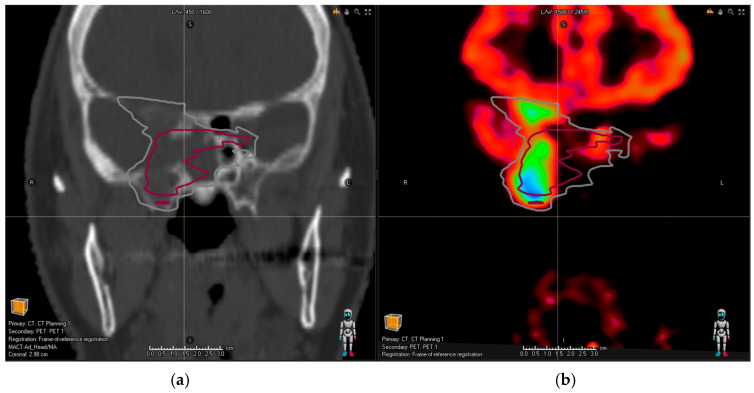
Planning CT image (a) of a patient with an aggressive pituitary adenoma. Red contour shows the common MRI volume of all observers; (b) the addition of PET/CT revealed further bone infiltration into frontobasis (difficult to identify based on structural imaging alone as the patient had co-existing osteoporosis)—the gray contour representing common PET/CT and MRI volume takes this finding into account.
